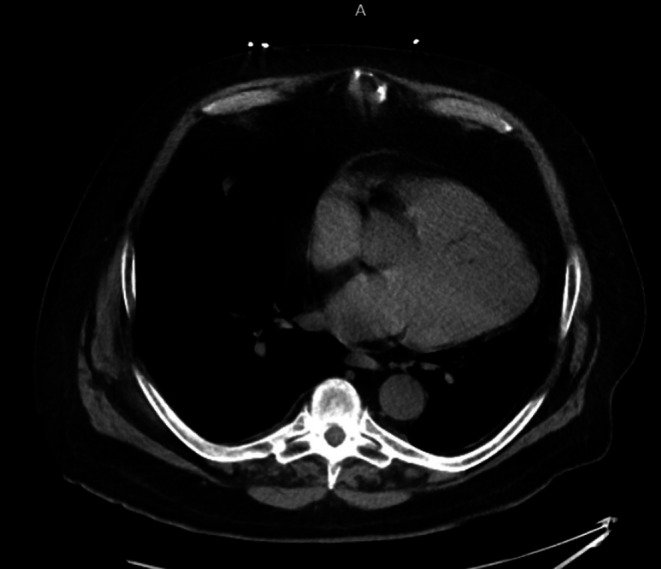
CT chest without contrast.
